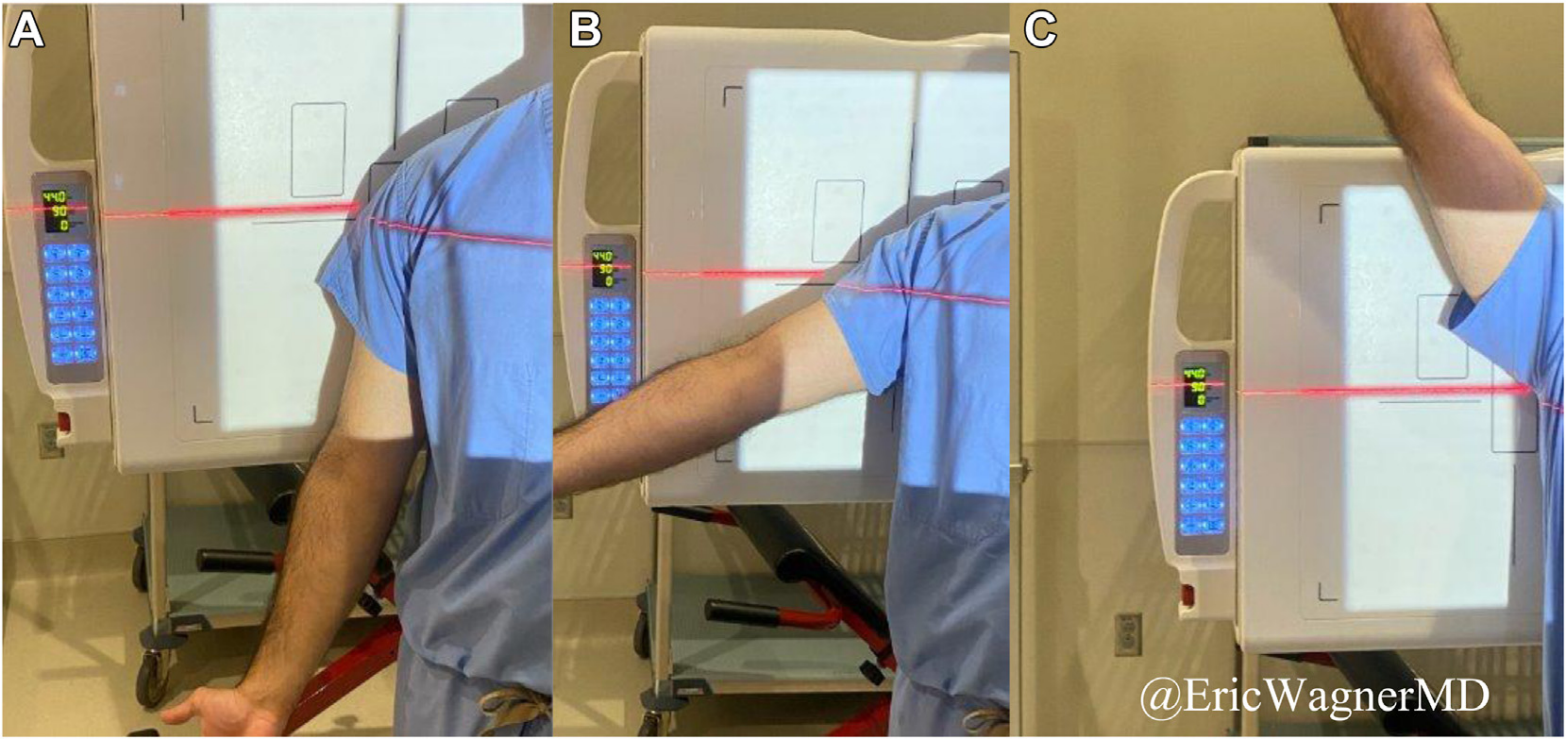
Procedure for capturing DDR images (A-C). Patients are positioned in a Grashey view with the posterior shoulder and scapula in contact with the back of the DDR machine. Each patient was instructed to stand upright, stationary, and avoid axial rotation, while following a video providing coordinated instructions on how to perform the motion, standardizing for forearm supination. Patients begin with their arm at rest (A) and gradually abduct their arm to maximum abduction (C). *DDR*, dynamic digital radiography.
